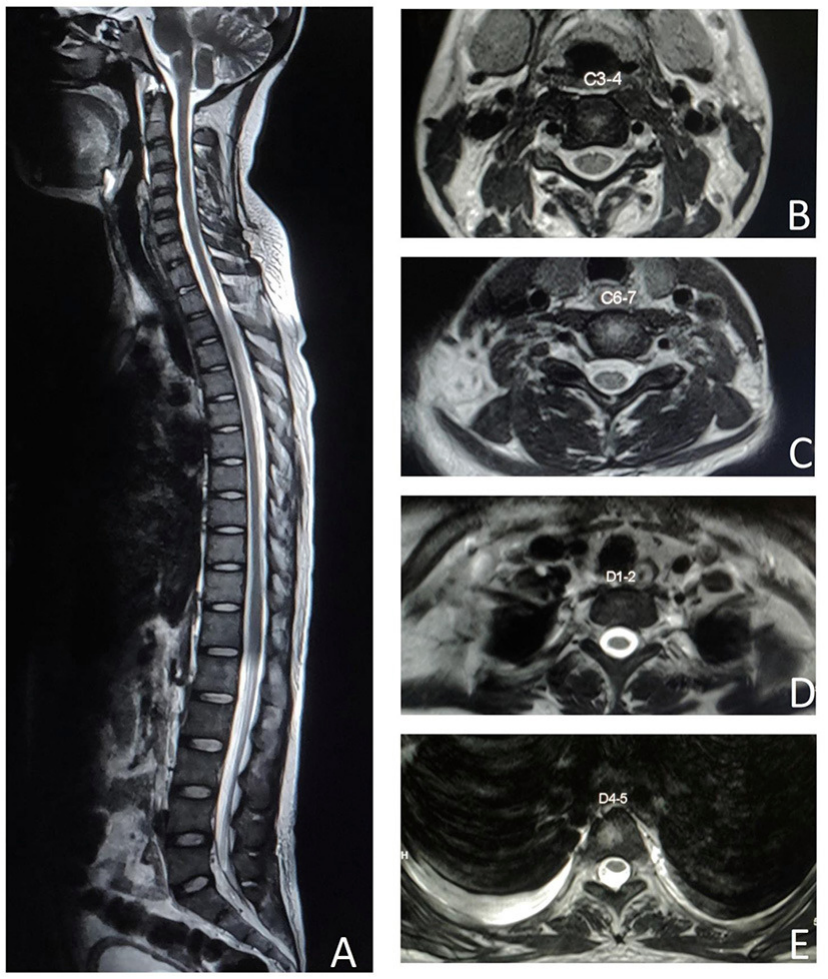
MRI spine T2 weighted image shows no spinal cord signal changes in the sagittal section (A), cervical axial sections at C3–4 (B), C6–7 (C), dorsal axial sections at D1–2 (D), and D4–5 (E).
